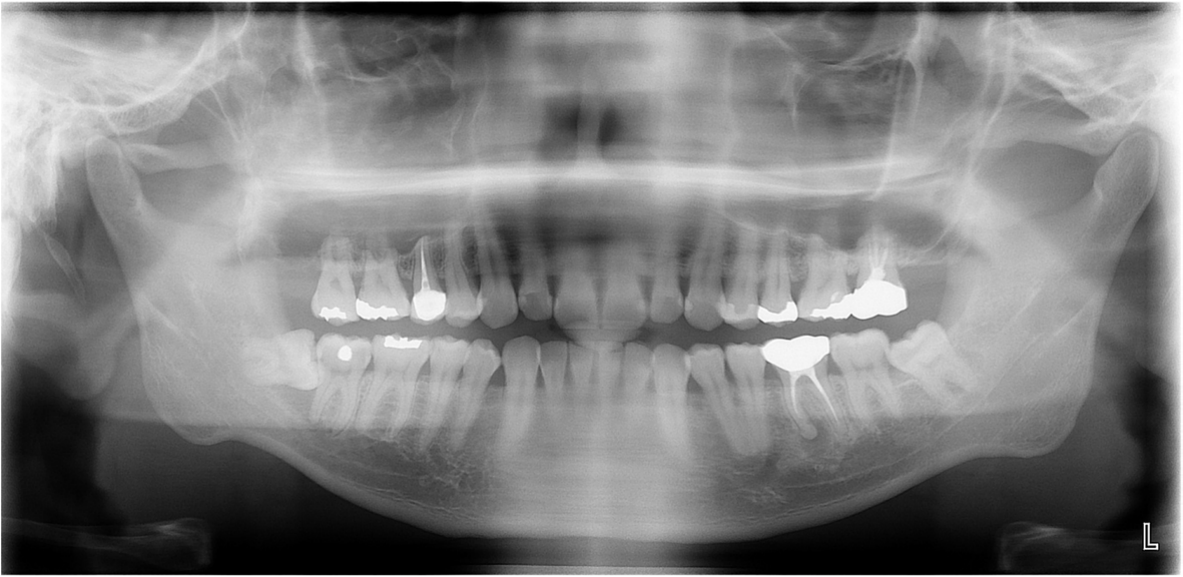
The panoramic radiograph of a 42-year-old male patient with ameloblastoma in the right maxilla
